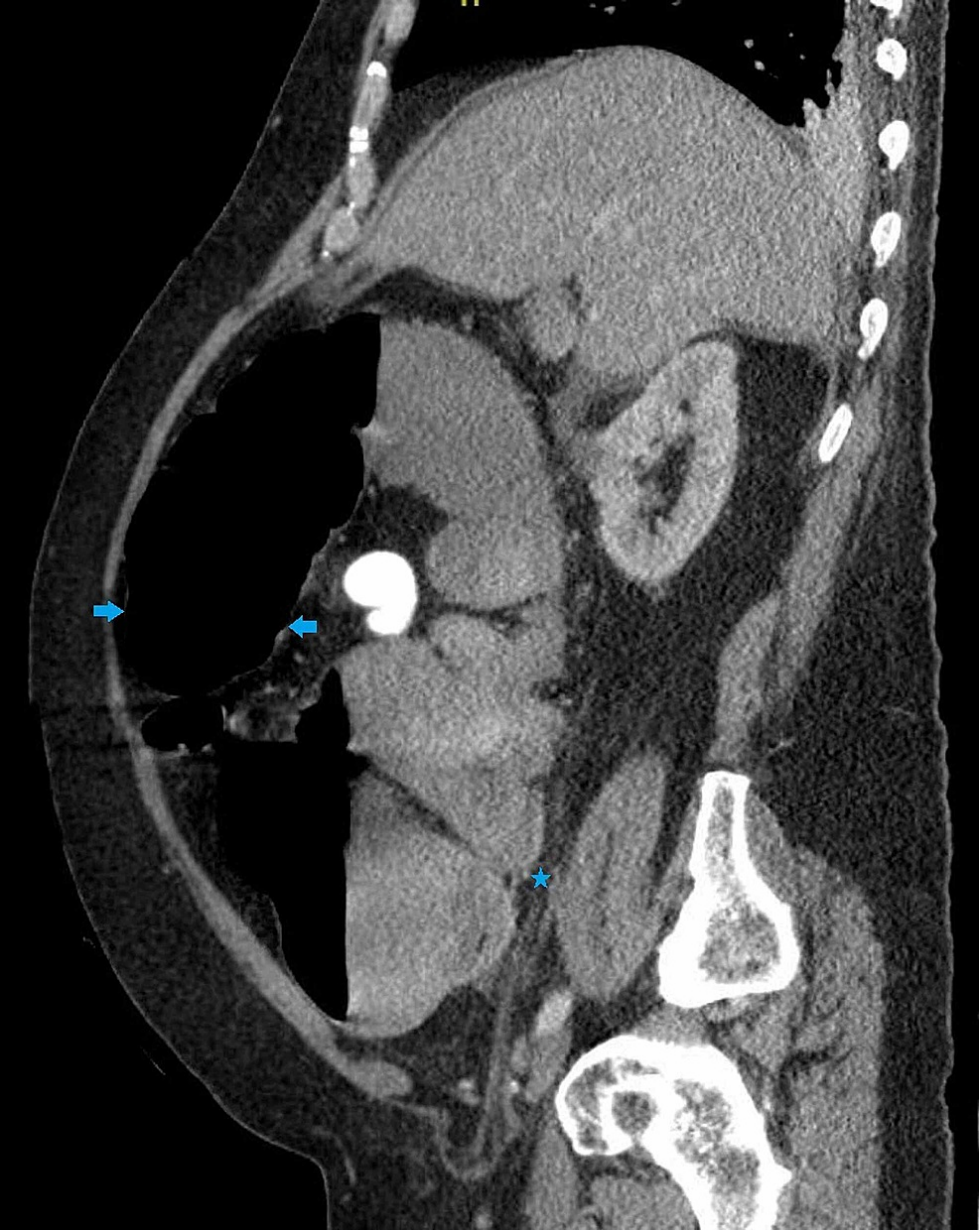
A repeat CT scan of the abdomen demonstrating colonic dilatation and wall thickening involving the distal transverse and descending colon, with pericolonic infiltrative changes representative of inflammatory colitis CT: computed tomography
